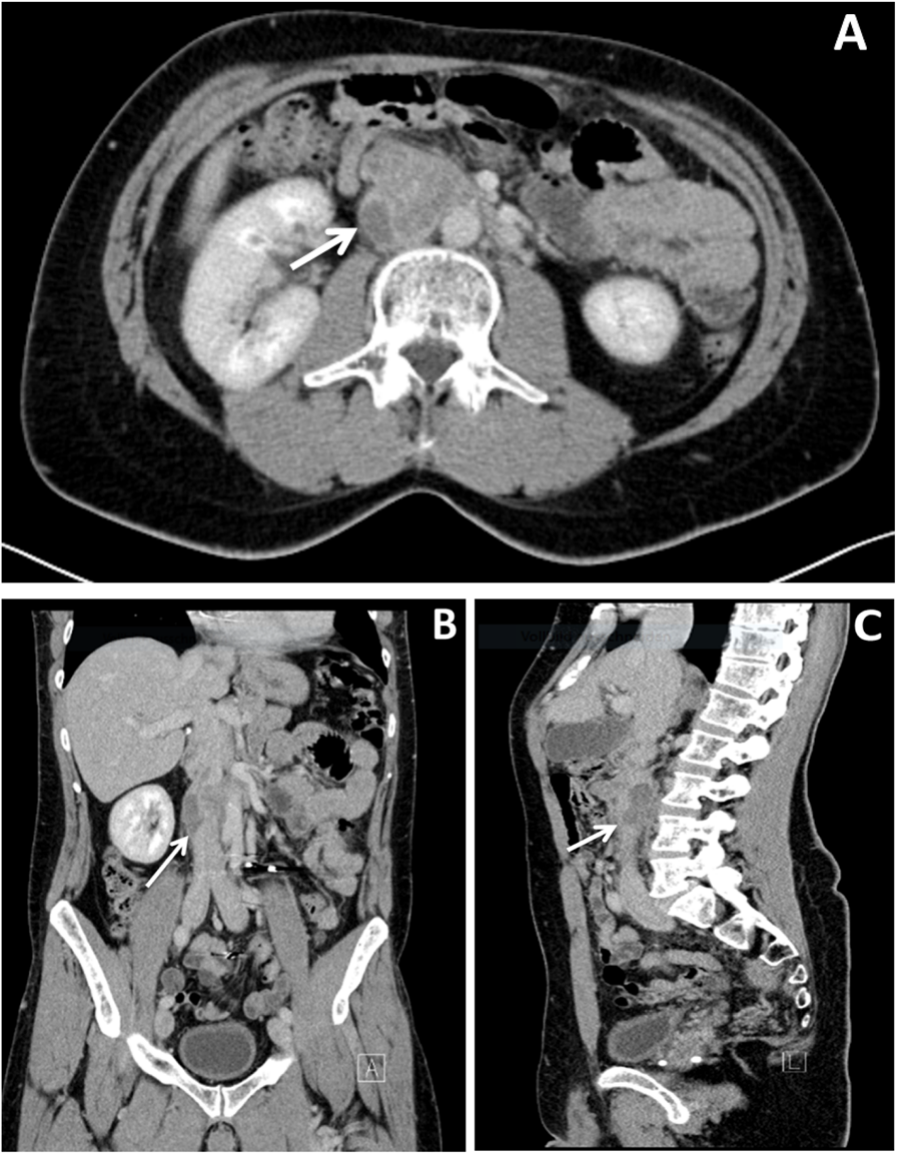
CT scan demonstrating a lesion (41.7 × 30.1 × 57.8 mm) with IVC infiltration and thrombus within the IVC (white arrows). a Axial. b Coronal. c Sagittal
